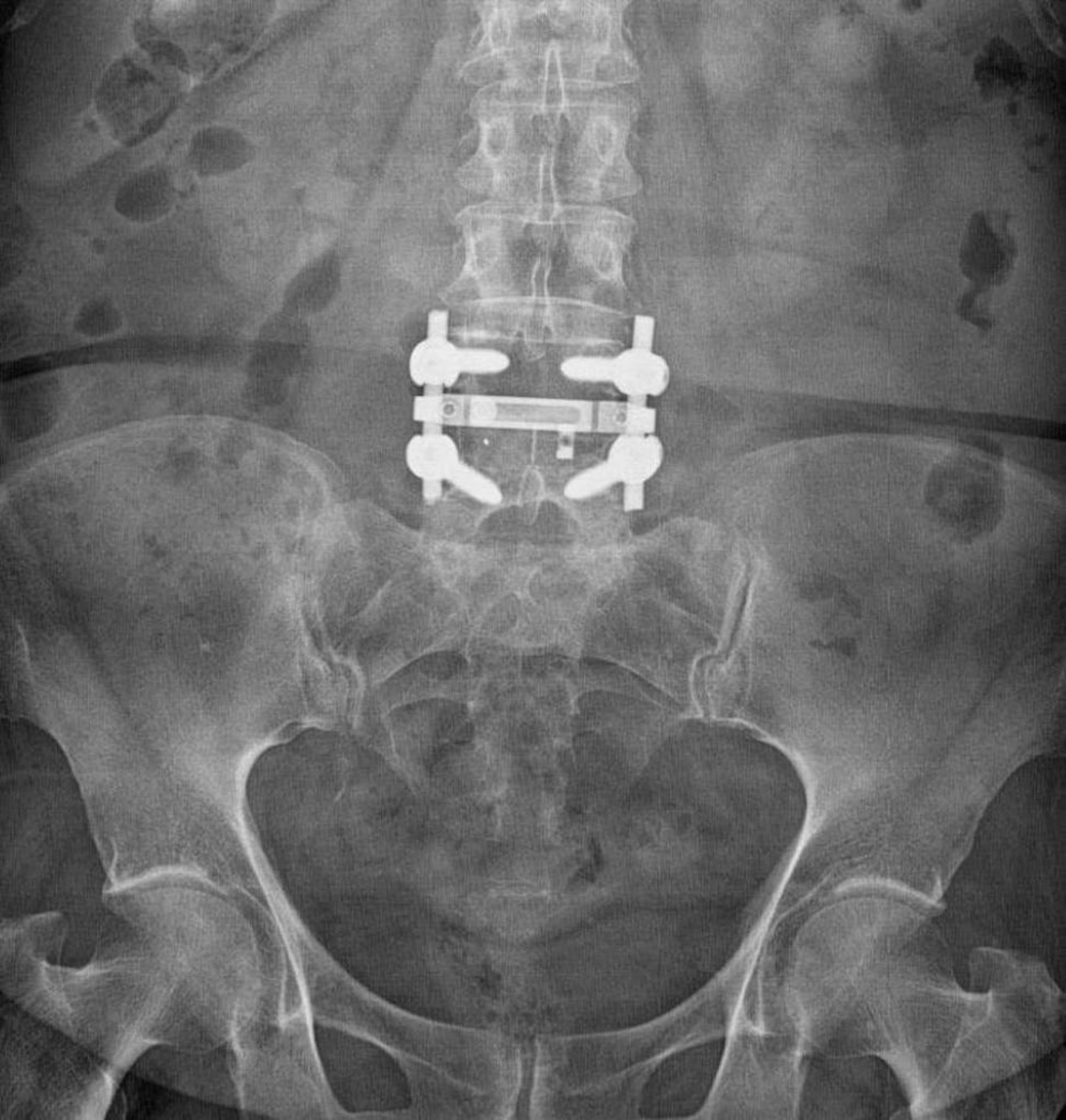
Postoperative radiograph of a patient with bilateral radiculopathy after a transforaminal interbody fusion procedure.
